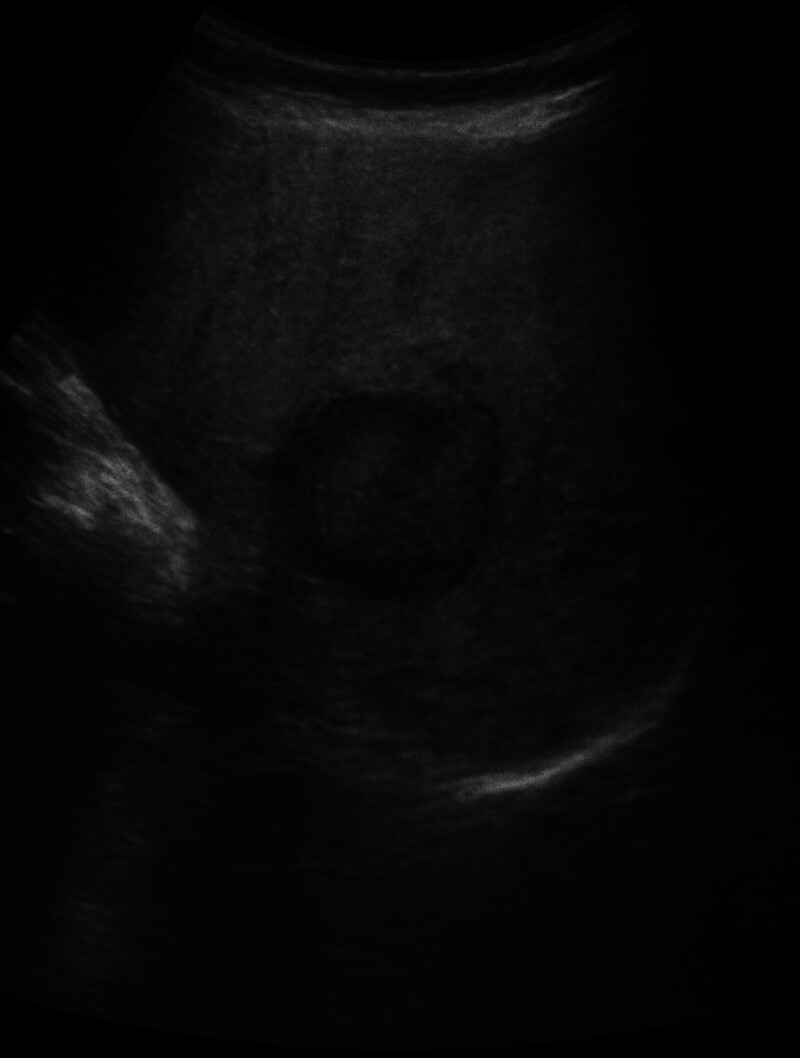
Abdominal ultrasound showing a 2 cm hepatic adenoma.
